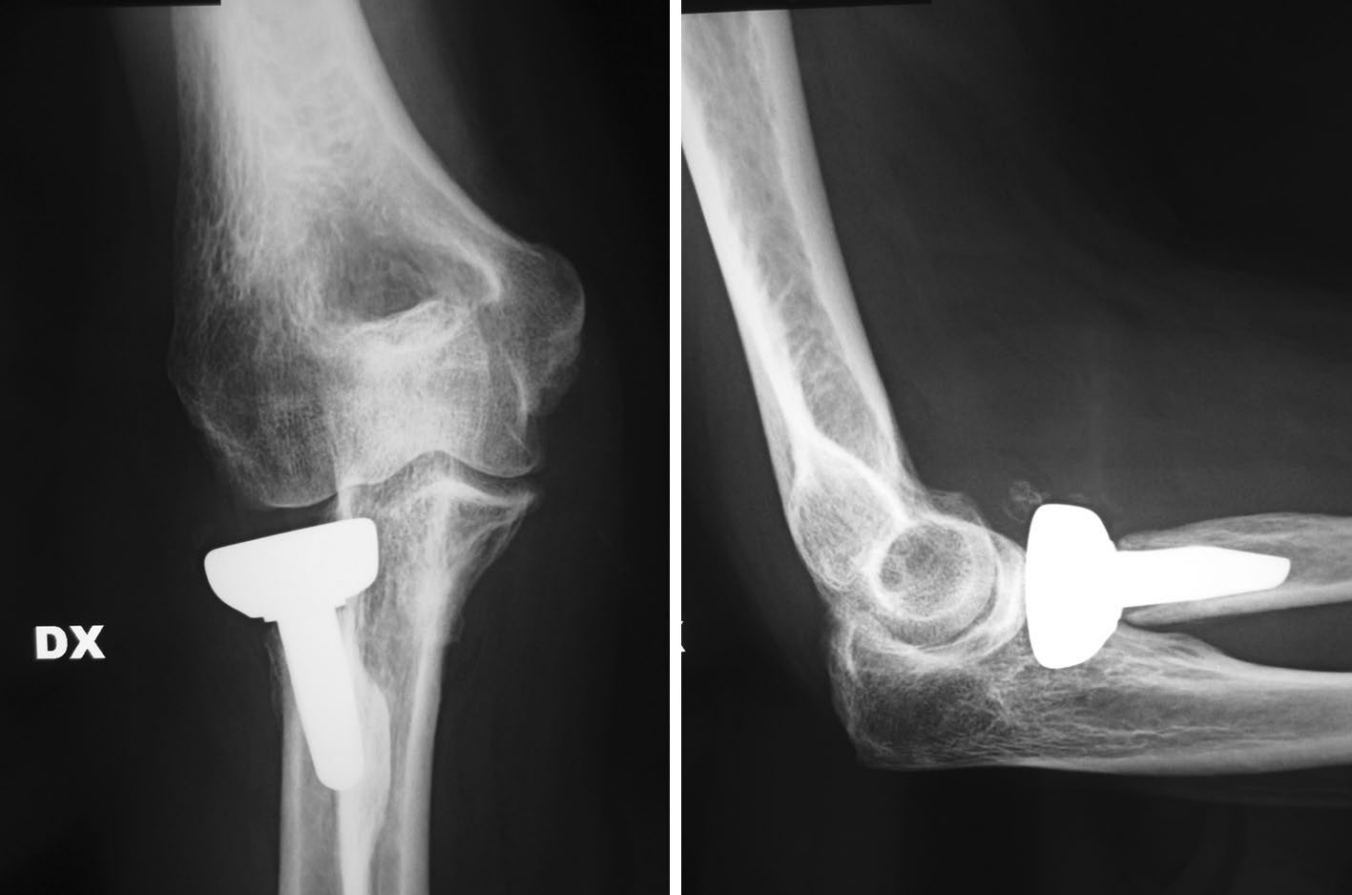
Two months X-ray follow-up of radial head prosthesis implanted for radial head malunion
